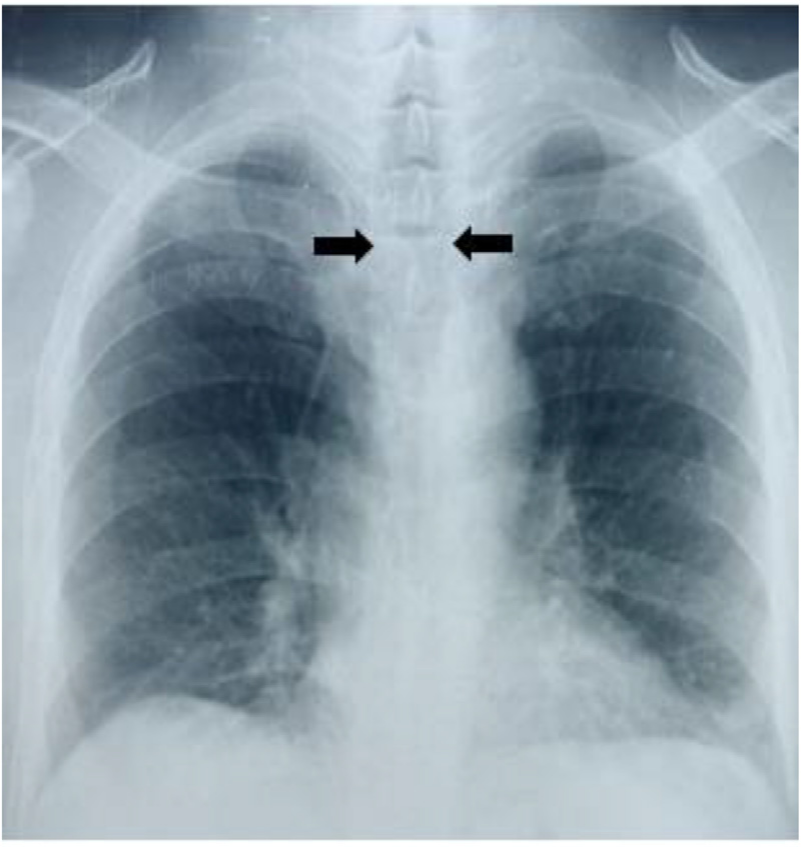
Frontal chest X-ray: marked enlargement of tracheal clarity with thoracic distension.
